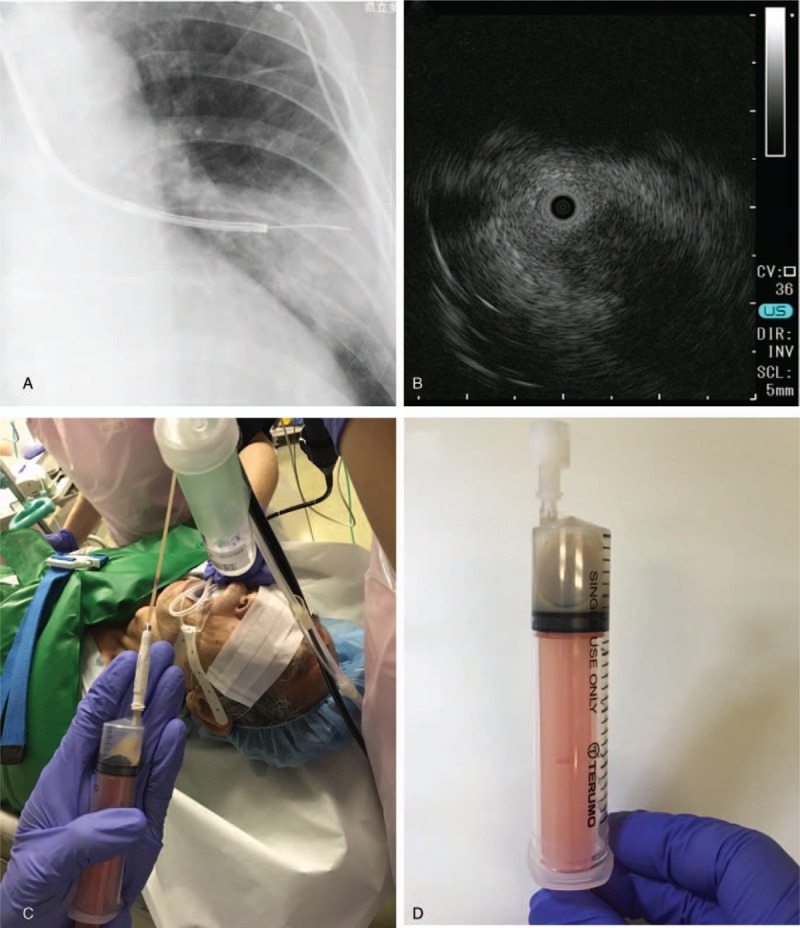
(A) Fluoroscopic image of the guide sheath placed in the shadow. (B) Ultrasound imaging in which the probe was placed within the lesion using radial EBUS. (C) Pus being collected into a syringe through the guide sheath during bronchoscopy. (D) A total of 4–5 mL of gray turbid pus was collected.
